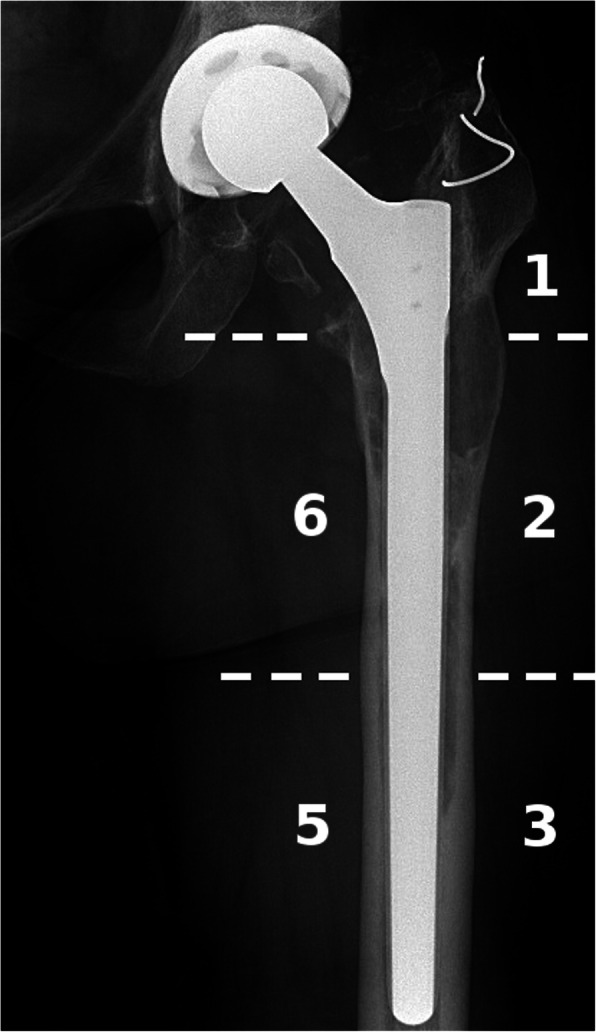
Example for evaluation of Gruen zones on AP radiograph (Patient 18)
